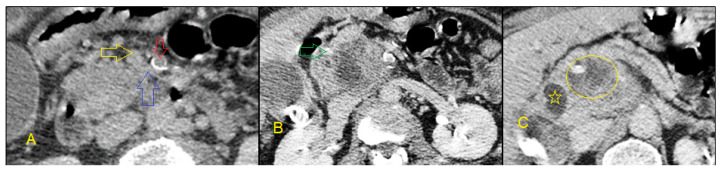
A–C: CT shows a pancreatic head mass with central necrosis (circle, C). Additional findings include no calcifications to support underlying chronic pancreatitis, no ductal dilatation, peripancreatic fat planes are not maintained (blue arrow, A) and superior mesenteric artery (SMA) (red arrow, A)/superior mesenteric vein (SMV) (yellow arrow, A) ratio is ≥ 1. The gastroduodenal artery (green arrow, B) is encased by the mass. Dilated common bile duct (star, C). Overall, there are at least three signs suggesting the diagnosis of malignancy.
